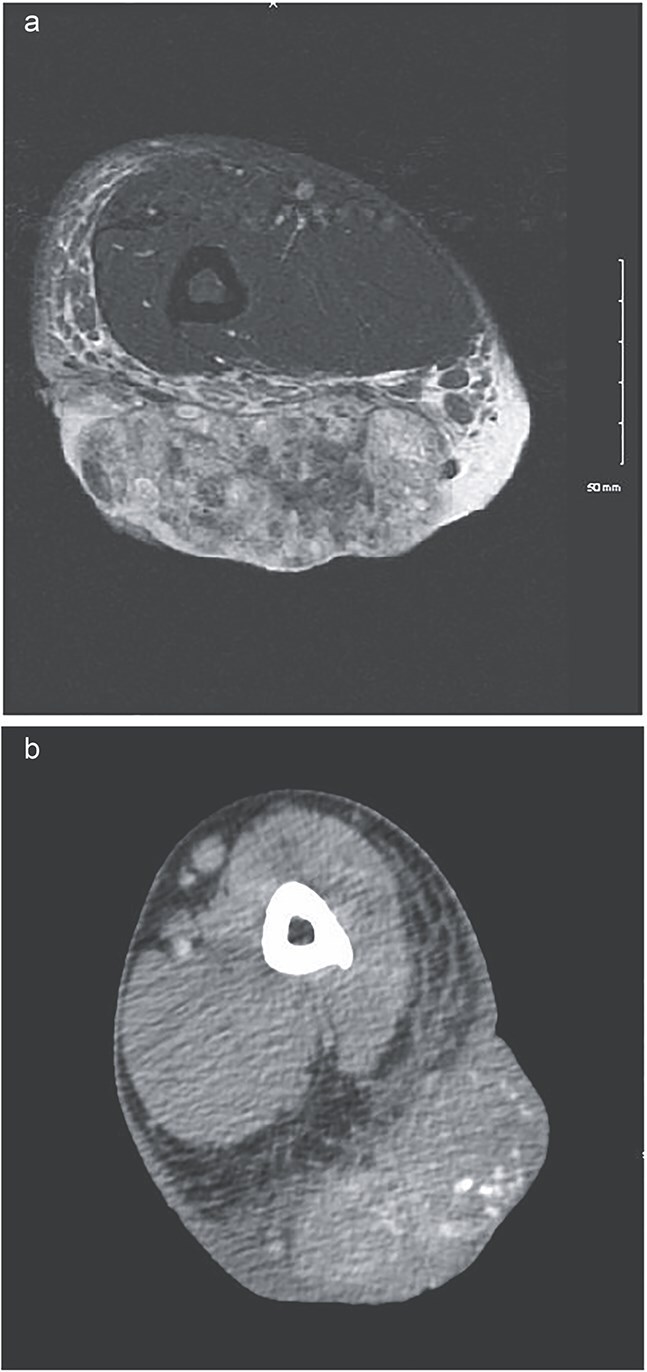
(a) MRI showing low signal on T1 and heterogeneously increased in signal on T2 sequences. Extensive surrounding subcutaneous edema with involvement of the dermal layer, as well as mild edema along the anterior fascia of the muscle. No definitive involvement of the muscle compartments noted, no involvement of neurovascular bundle. Some enlarged epitrochlear lymph nodes seen within the medial aspect of the elbow, with concern for possible local metastatic disease. (b) CT upper extremity demonstrating calcified arm lesion with vascularity supplied from branches of the proximal brachial artery. Involvement in the dermis and subdermal fat.
